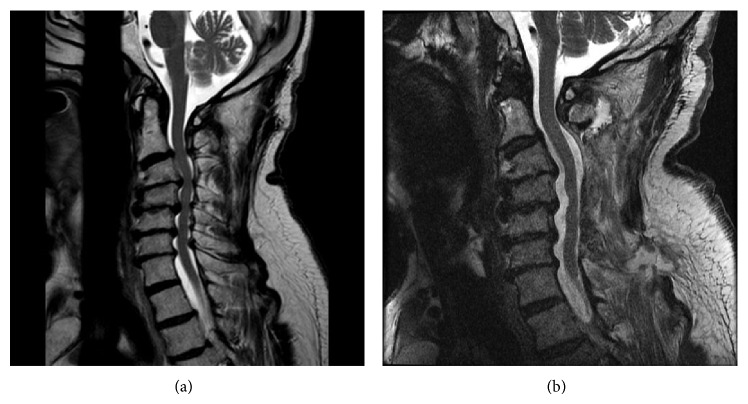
Pre- and postoperative T2-weighted sagittal MRI (static). Postoperative imaging in the neutral position appears to show satisfactory decompression.
